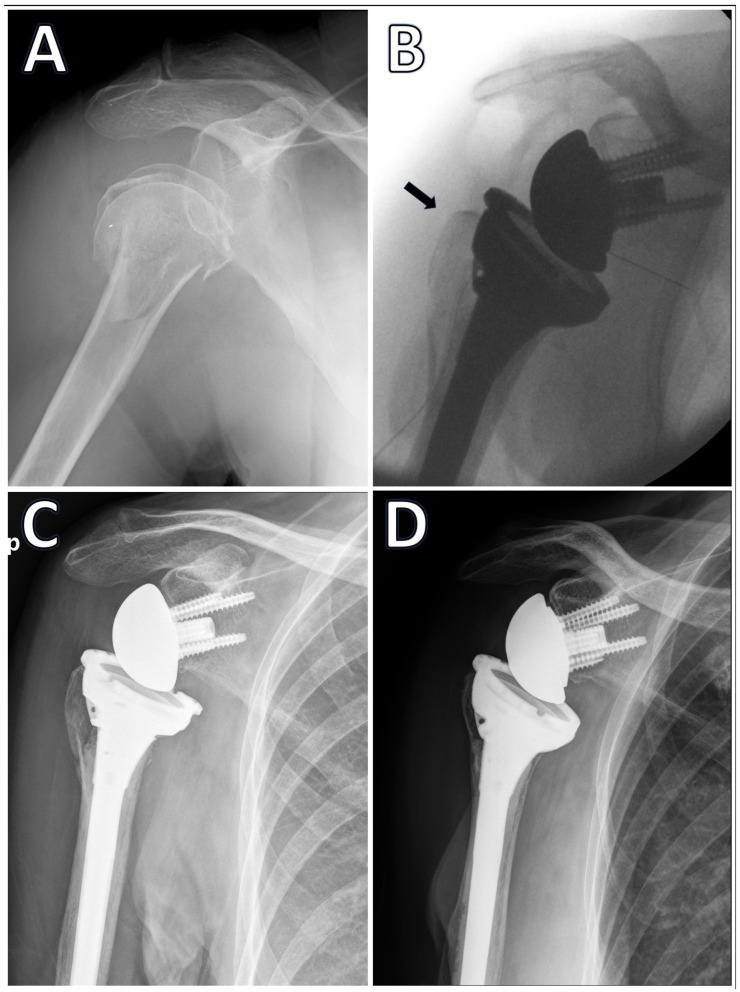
A 77-year-old female presented with a 4-part displaced fracture of the proximal humerus (A); she underwent RSA using a Depuy Delta Xtend shoulder system, which was implanted with bone cement. Intraoperative fluoroscopy shows good reduction in the greater tuberosity (black arrow) (B); an AP radiograph at 4 weeks after surgery shows no displacement of the greater tuberosity (C); at 15 months after surgery, there is a complete healing of the greater tuberosity in continuity with the humeral shaft (D).
